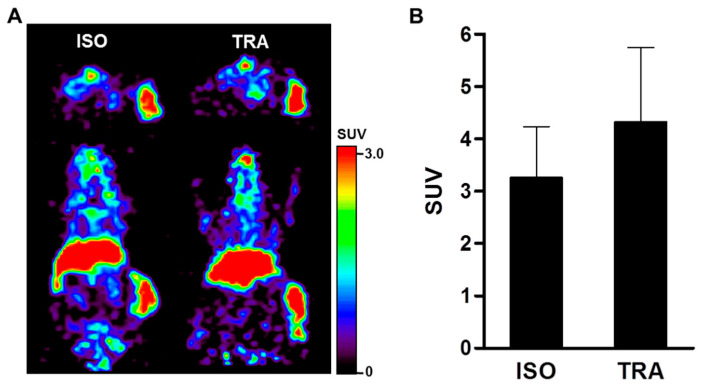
Immuno-PET imaging of ^89^Zr-DFO-pertuzumab by trastuzumab treatment in JIMT-1 breast cancer xenograft model. (A) To evaluate the dynamics of HER2 expression level by treatment of isotype (ISO) and trastuzumab (TRA) using ^89^Zr-DFO-pertuzumab, Immuno-PET imaging was performed in the JIMT-1 breast cancer xenograft model. Immuno-PET images were acquired 7 days after injection of ^89^Zr-DFO-pertuzumab. (B) JIMT-1 tumor uptake of ^89^Zr-DFO-pertuzumab as quantified using ASIPro display software. All images and quantitative data were expressed as a standardized uptake value (SUV).
